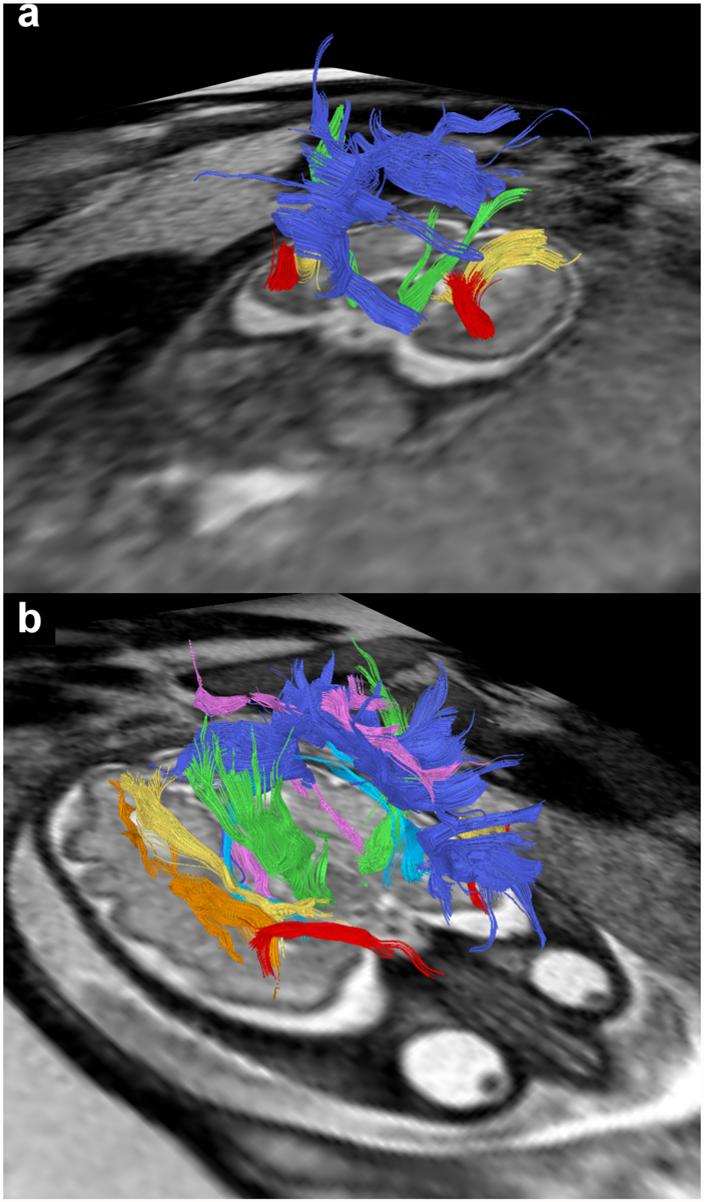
In utero tractography of association and limbic fiber tracts in subjects 1 (a) and 20 (b) (20 and 33 GW respectively) and their relation to the corpus callosum (dark blue) and corticospinal/ corticopontine fibers (green). Fiber tracts are projected on axial T2-weighted images. Visualized association fiber tracts include the UF (red), the IFOF (yellow), the ILF (orange) and the cingulum (pink). The limbic fiber bundle of the fornix is color-coded in light blue.
